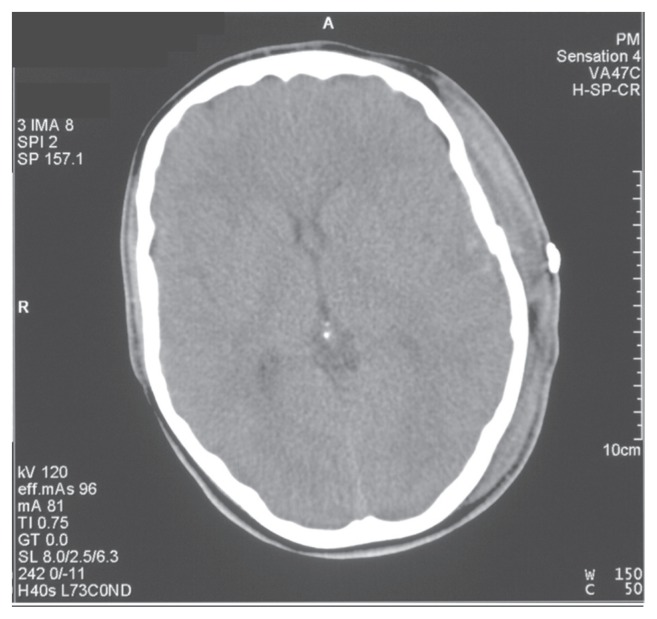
Post operative CT scan, the haematoma has been evacuated.
